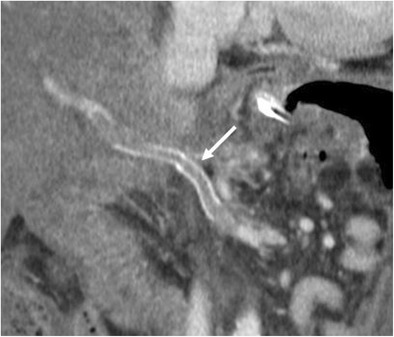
Portal vein thrombosis. CT curvilinear coronal reconstruction image shows the presence of a massive thrombosis of the superior mesenteric and portal venous axis (white arrow) following a DCP with a resection of the portal vein and prosthetic reconstruction
